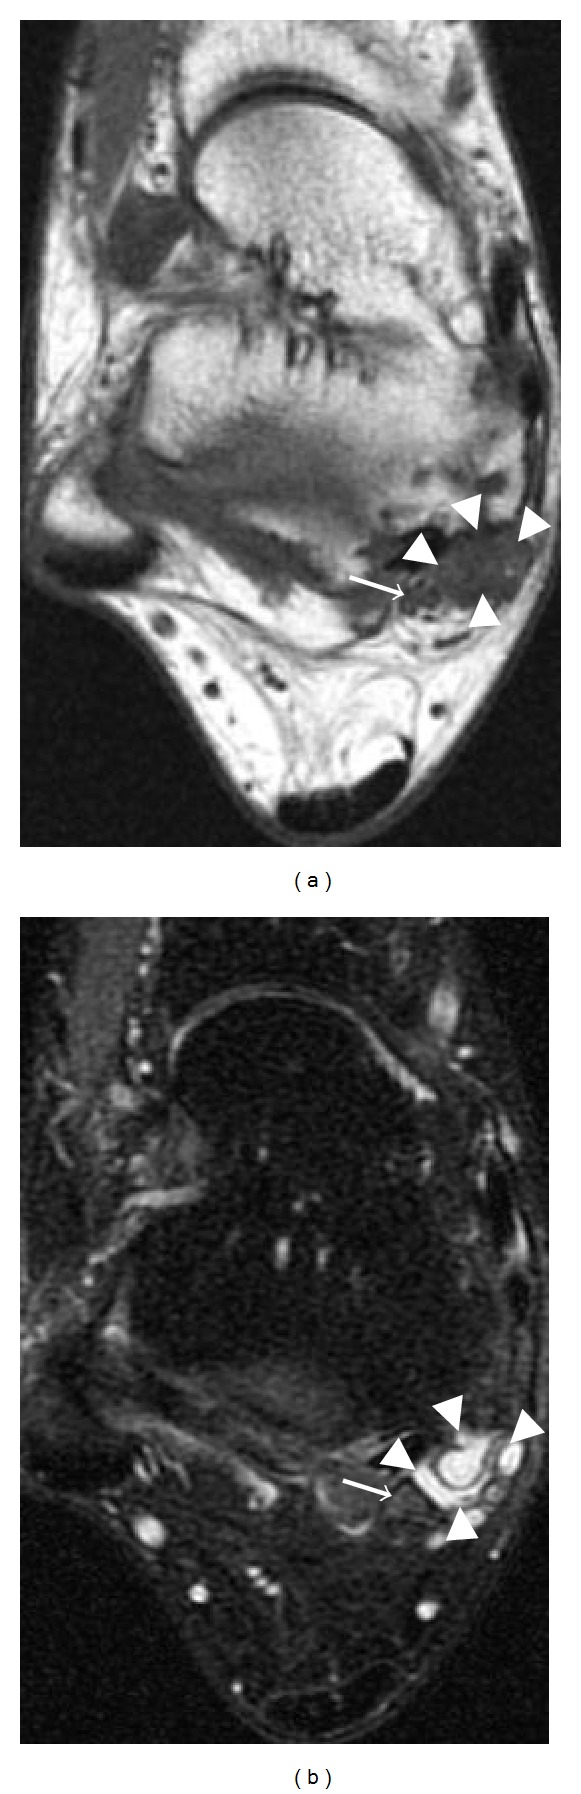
Tarsal tunnel syndrome caused by a ganglion cyst in a 32-year-old patient. Axial T1-weighted (a) and T2-weighted (b) fat-saturated images show a multilobulated cystic structure (arrowheads) within the right tarsal tunnel. Note the adjacent tibial nerve (arrows).
